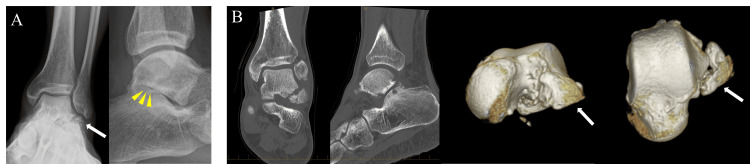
Plain radiograph and CT findings. A: Plain radiographs at the time of visit to our clinic. Nonunion of fracture of the lateral process of the talus (FLPT) and subtalar joint space narrowing were observed. B: CT showed a large bone fragment, subchondral bone sclerosis, and cystic lesion at the subtalar joint. C: 3D-CT showed a large fragment of fracture of the lateral process of the talus.
